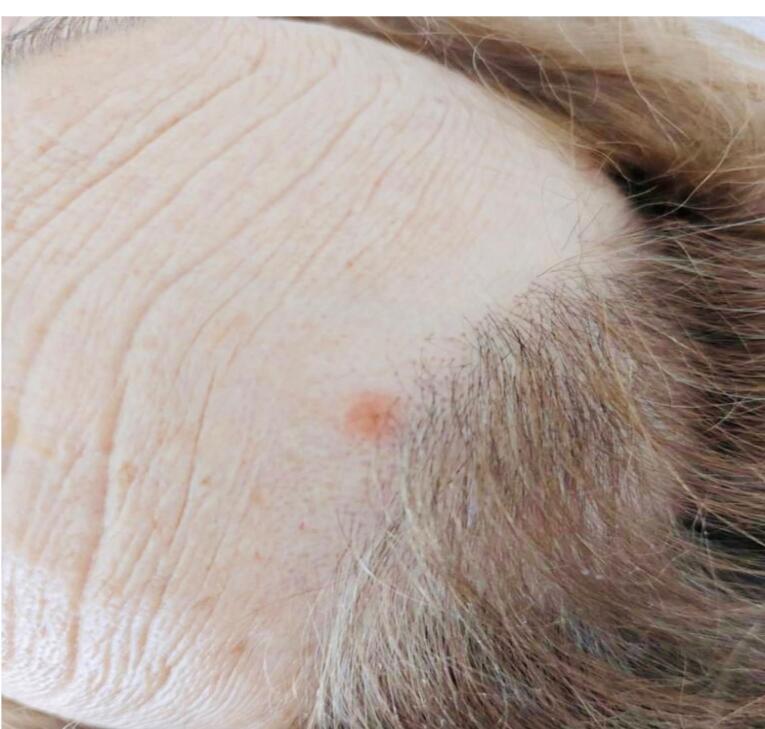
Punch biopsy of the skin lesions and chest X-ray were performed with presence of interstitial infiltrate in the right pulmonary base.
